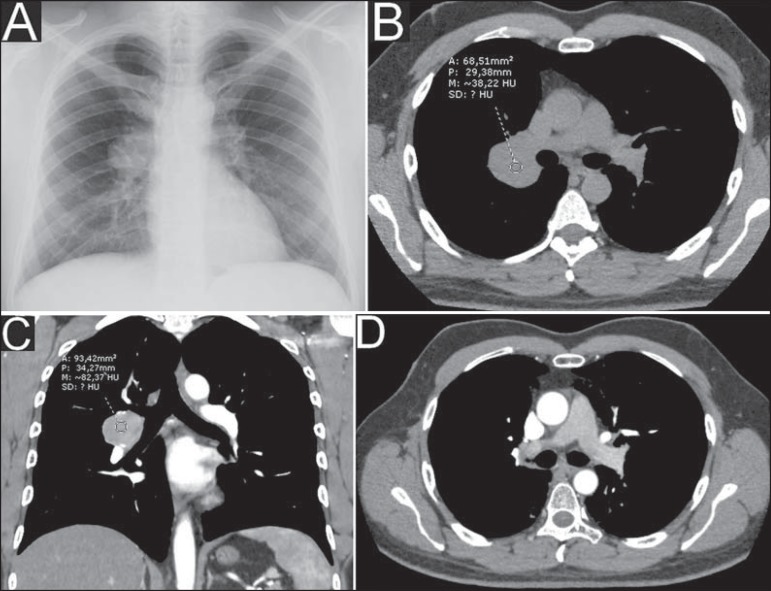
Chest radiography (A) showing ovoid opacity in the right hilar
